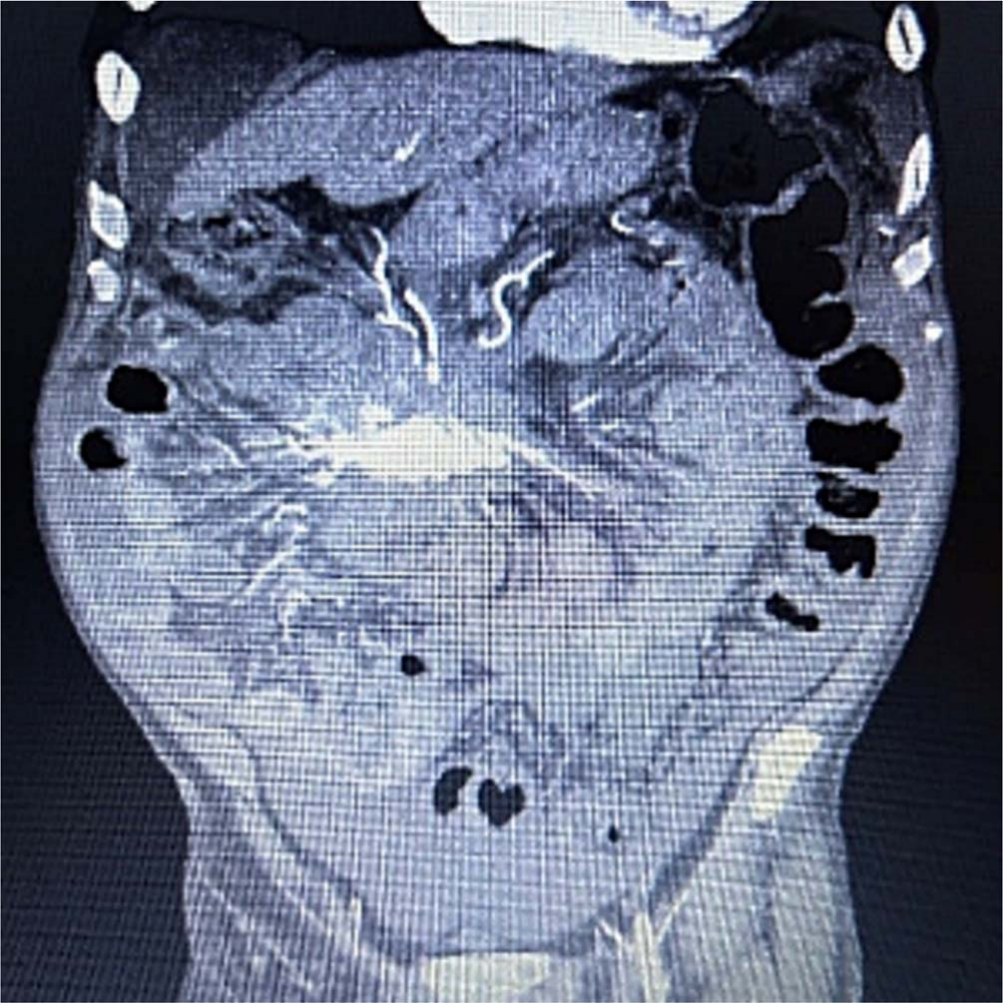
Anterior–posterior view of CT abdomen/pelvis scan showing large, central calcified mesenteric mass, Case 1.
